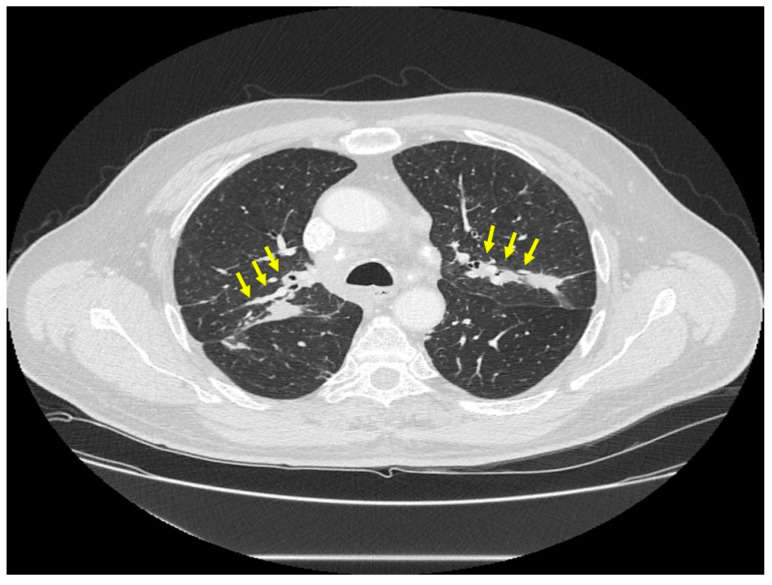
Chest CT scan image of a patient with pulmonary sarcoidosis, demonstrating lung opacities from granulomatous inflammation around airways (arrows).
